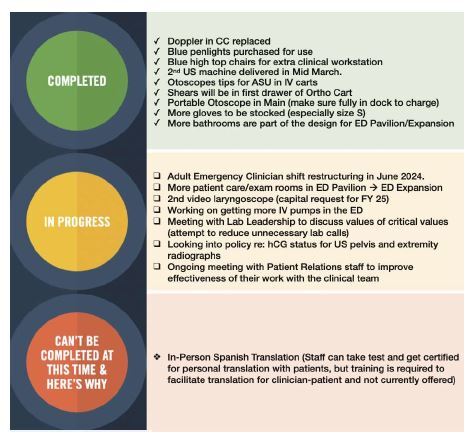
“Pebbles in shoe” project traffic stoplight report Winter Quarter 2024. *CC*, critical care; *ASU*, Ambulator Surgical Unit; *US*, ultrasound; IV, intravenous; *ED*, emergency department; *Ortho*, orthopedic; *FY*, fiscal year; *hCG*, human chorionic gonadotropin.
